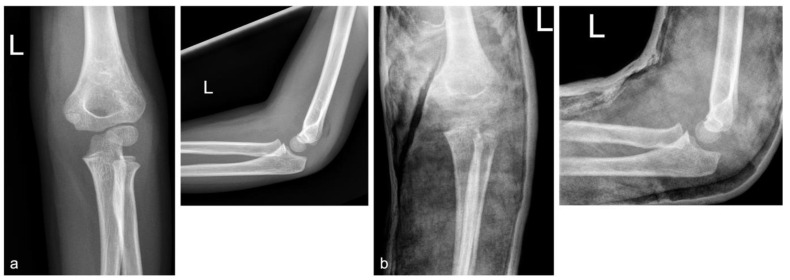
The course of a conservatively treated type II supracondylar fracture of the left (L) arm of a 5-year-old girl who fell from a standing position. (a) Initial anterior–posterior (a.p.) and lateral X-rays showing a type II fracture according to the Von Laer classification. (b) A.p. and lateral X-rays within the upper-arm cast (for three weeks) showing the fracture in an unchanged position (approximately 4 days after injury for exclusion of a secondary dislocation). Patient presented full range of motion and no deficits two weeks after cast removal.
